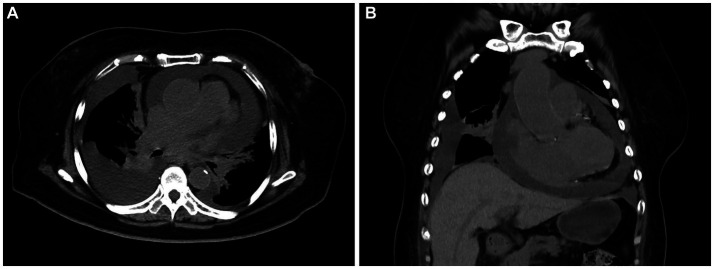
Computed tomography (CT) images of primary cardiac lymphoma (PCL). Transverse (A) and coronal (B) images revealed multiple nodular and clumped slightly hypodense foci in the heart and pericardium, along with effusions in the pericardium and pleural cavity.
